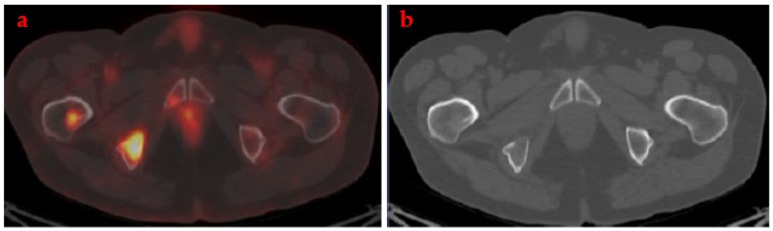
FDG-PET/CT showed metastatic bone lesions (a) undetected by CT (b).
